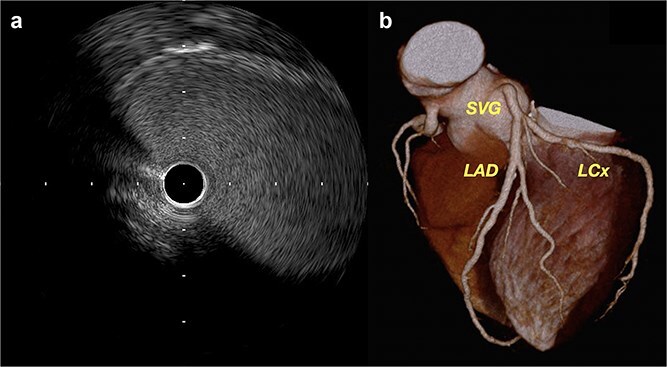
(a) Postoperative IVUS demonstrating a widely patent SVG with no stenosis. (b) Follow-up coronary CT at 6 months showing excellent graft configuration.
